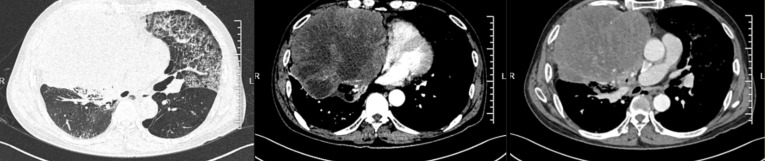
Chest computed tomography (CT) before chemoimmunotherapy (July 2023).
